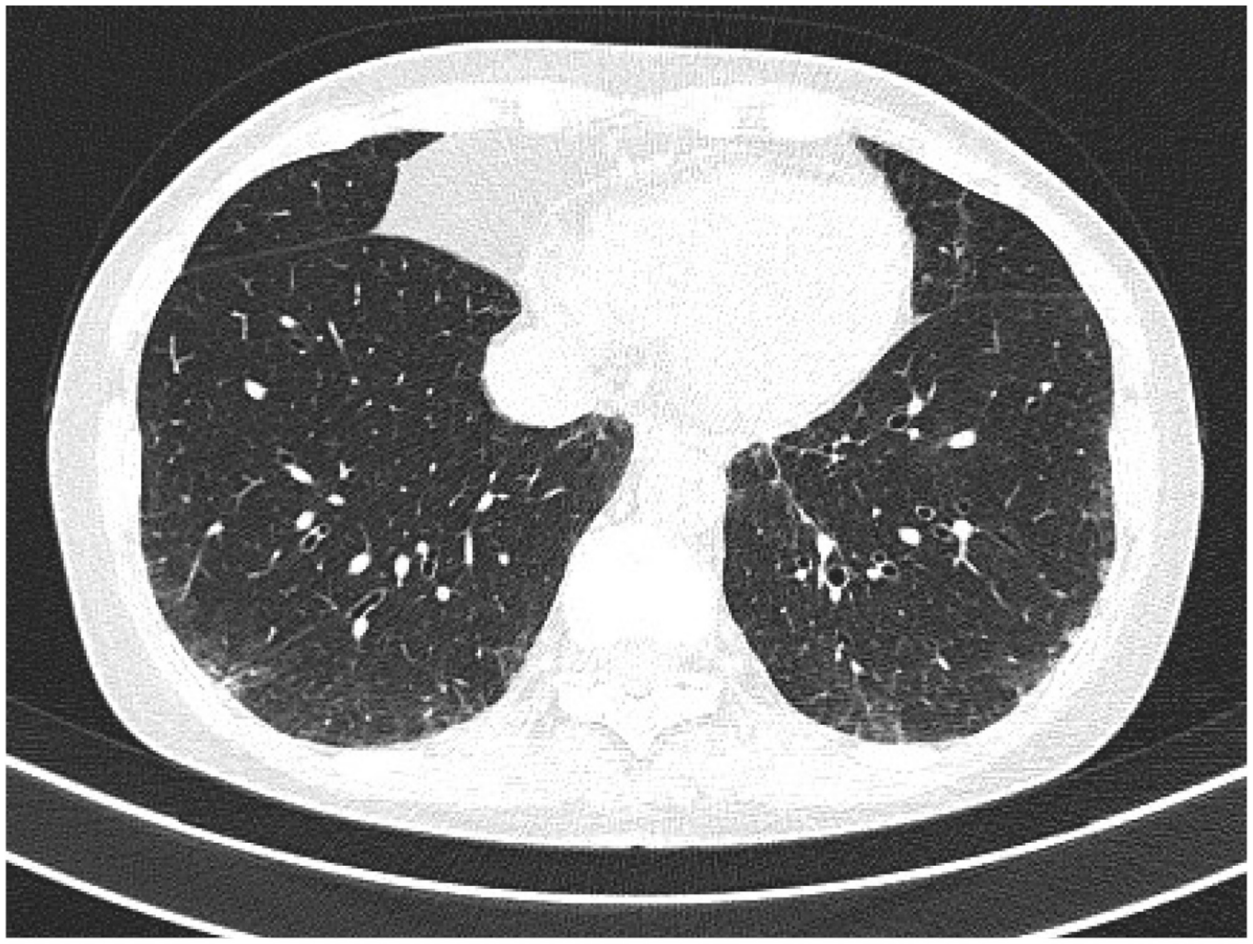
Bronchiectasis (BR) on HRCT in a patient with RA.
